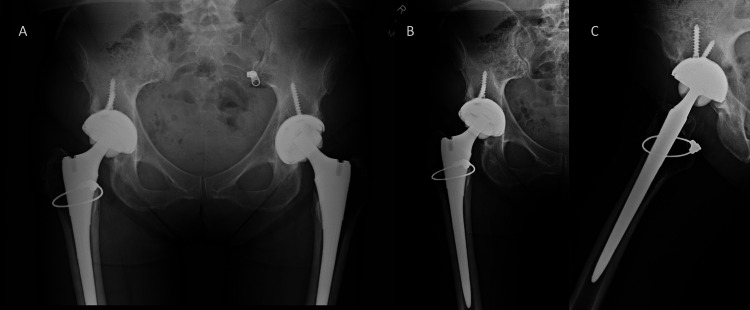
Preoperative anteroposterior (A) radiograph of the pelvis and anteroposterior (B) and lateral (C) radiograph of the hip showing a well-fixed and well-aligned right total hip arthroplasty with no signs of failure
